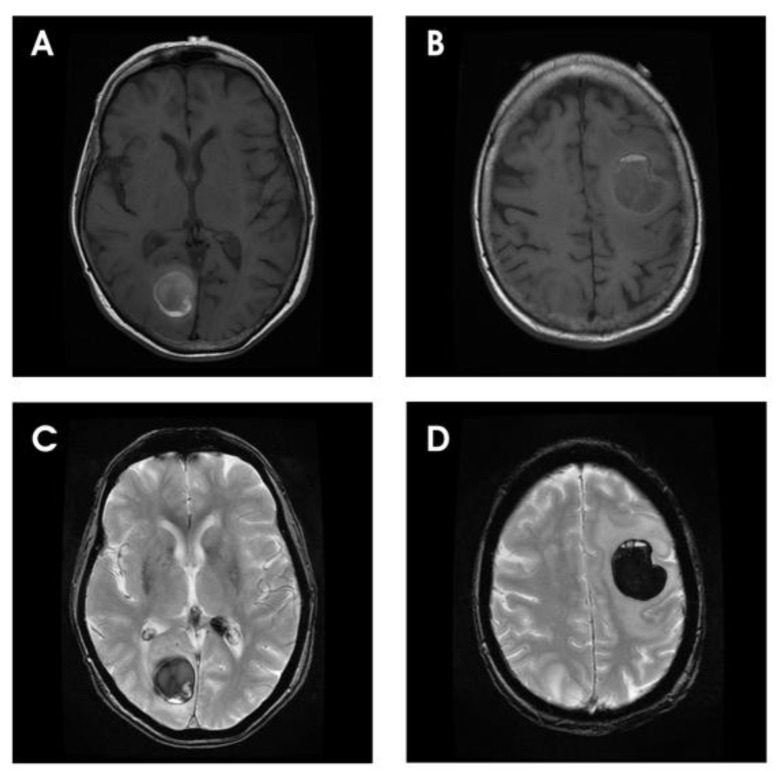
Axial MRI demonstrating multiple hemorrhagic lesions in a patient with metastatic melanoma. (A–B) T1 weighted images show two circumscribed lesions in the left frontal and the right occipital lobes. The mixed signal intensity within the lesion suggests the presence of hemorrhages of various chronicity. (C–D) T2* weighted sequence confirms the presence of hemorrhages as indicated by the areas of hypointensity. Additional hemorrhagic lesions are also shown in the left lateral ventricle and in the posterior third ventricle.
